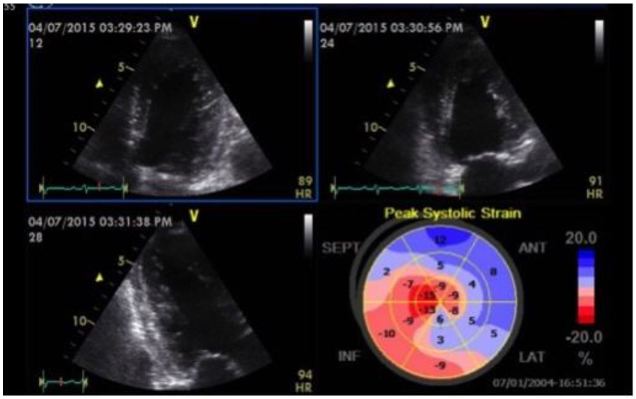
Baseline echocardiogram in a patient with acute hypertensive pulmonary edema. Quad view demonstrating apical 4-chamber, 2-chamber and long axis images with a bullseye map. Global longitudinal strain is −7.1%. There is significant dyskinesis in the anteroseptal, anterior and lateral segments.
